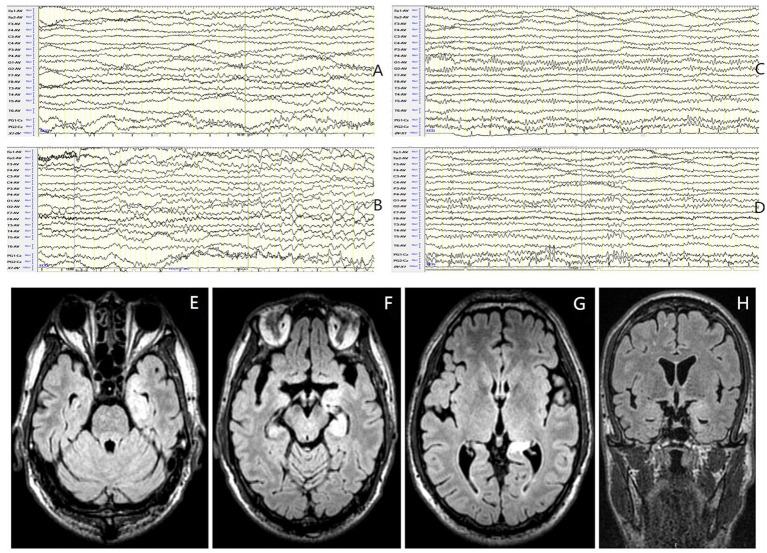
Case 39, male, 64 years old, who was diagnosed with anti-GABA_B_R encephalitis. (A,B) Twenty-one days after onset and before immunotherapy, EEG showed slow waves involving left temporal region in the background activity (A) and subclinical electroencephalographic seizure originating from left temporal region (B); (C,D) Ten days after immunotherapy, the background activity improved significantly (C) with slow waves in the left posterior region (D). (E–H) Before immunotherapy, brain MRI showed swelling of left hippocampus and T2 FLAIR hyperintensity in the bilateral hippocampus.
